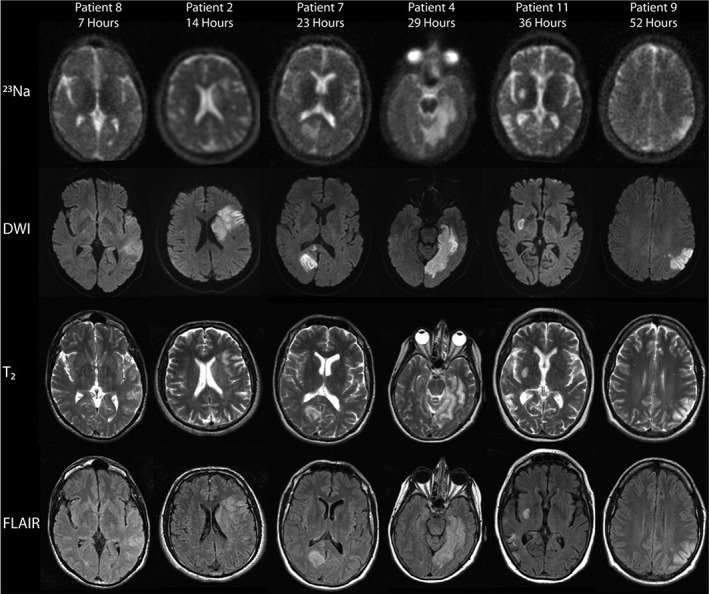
Representative sodium (^23^Na), diffusion‐weighted (DWI), T_2_‐weighted, and fluid‐attenuated inversion recovery (FLAIR) images from patients 7 to 52 hours after ischemic stroke onset. Areas of sodium signal intensity increase correspond to the lesions identified on DWI. The sodium signal intensity in the areas of ischemia qualitatively increased with time (figure reproduced from Ref. 112 with permission from Wiley).
